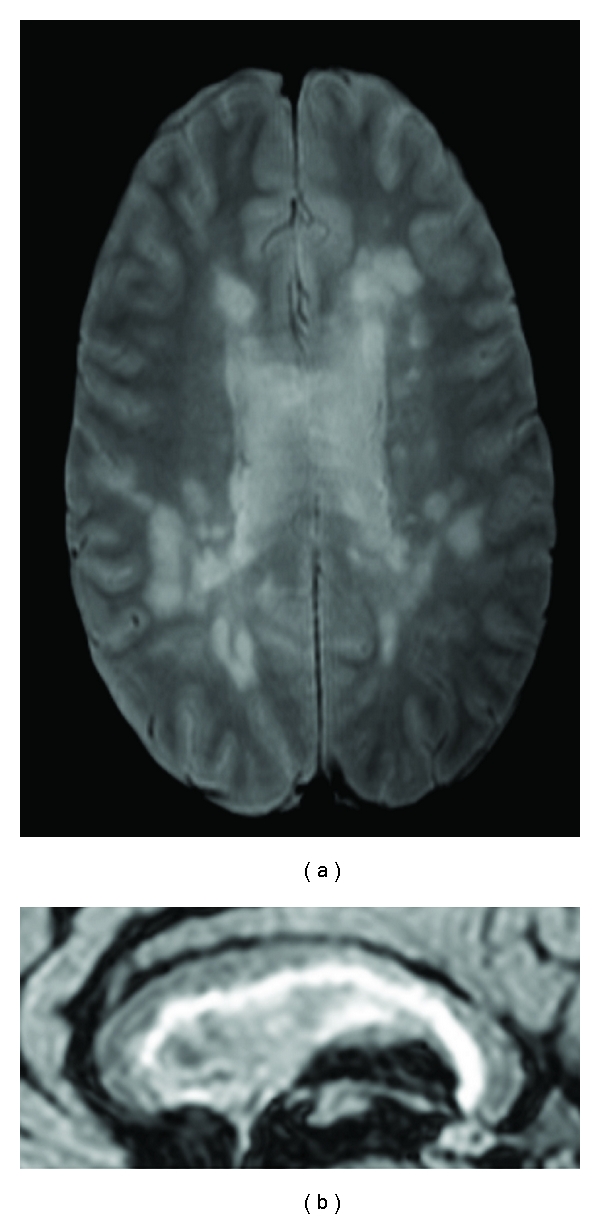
Female patient with high lesion load and no significant cognitive deficit. The PD axial image at the level of the body of the lateral ventricles and CC (a) shows lesions at the periventricular and subcortical white matter. The detail of mid-sagital FLAIR (b) shows the abundance of macroscopic lesion in the CC and callosal-septal interface.
